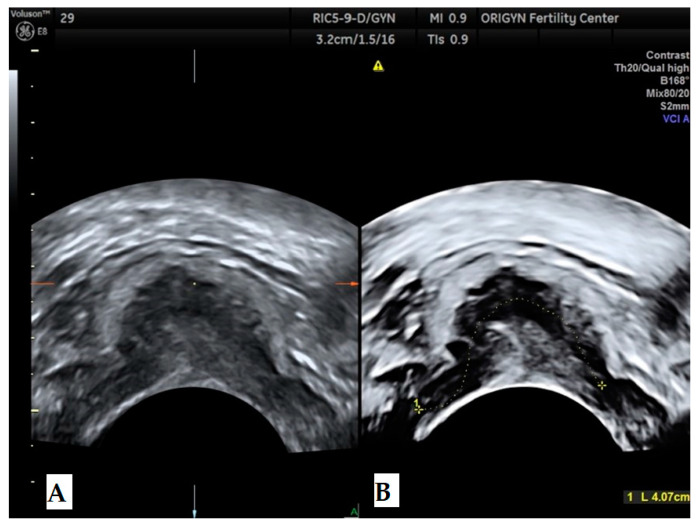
(A) Ultrasound image showing a deep infiltrating endometriosis (DIE) nodule with a regular outline (absence of “spikes”). (B) the same nodule is viewed in 3D with TUI (tomographic ultrasound imaging).
